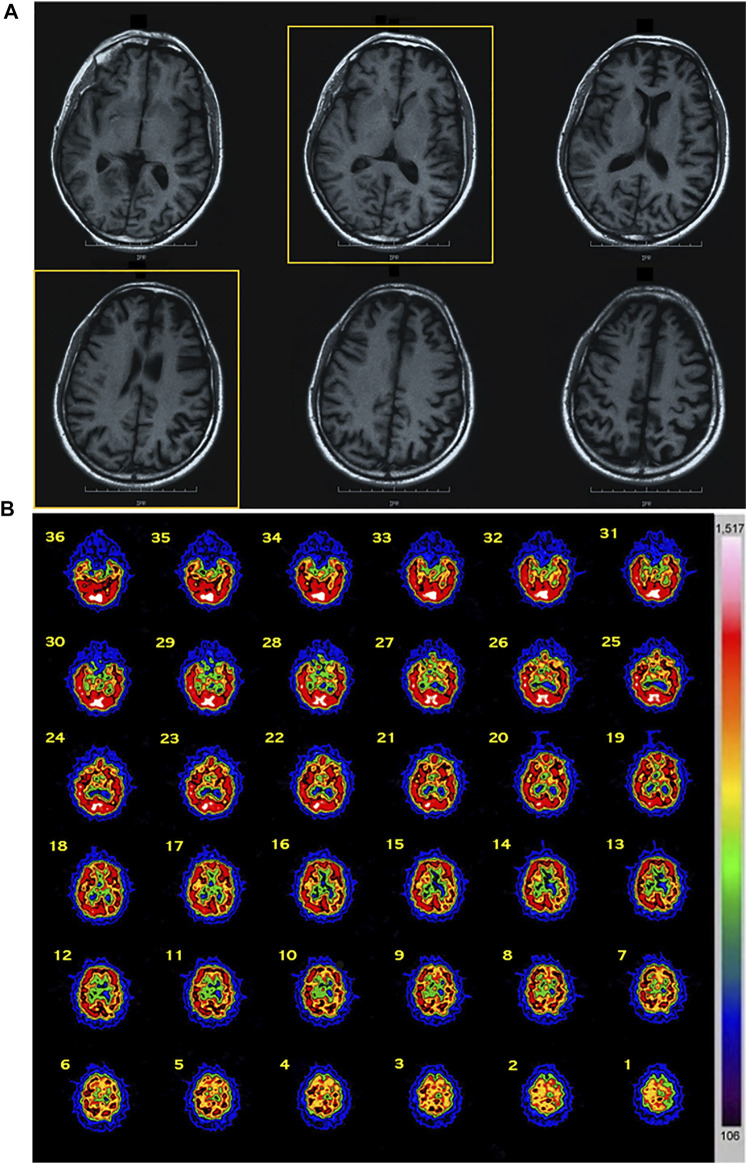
Imaging data of the proband. (A) MRI data: diffuse cortical atrophy prominent in frontal and temporal regions was present. (B) SPECT data: a hypoperfusion was observed in frontal and temporal convolutions of the left hemisphere and upper frontal circumvolution of the right hemisphere. Abbreviations: MRI, magnetic resonance imaging; SPECT, single-photon emission computed tomography.
